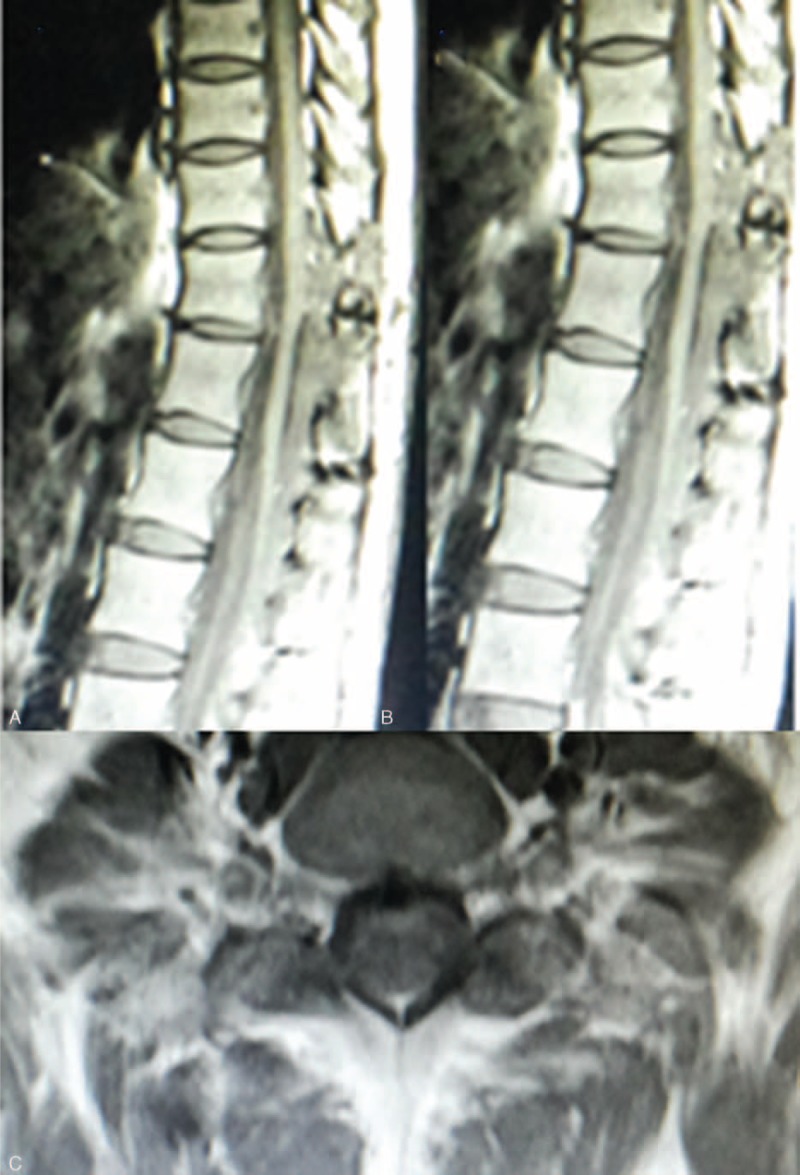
Postoperative sagittal T1-weighted (A), T1-weighted enhanced with gadolinium (B), and axial T2-weighted (C) magnetic resonance (MR) images demonstrated no mass residual.
